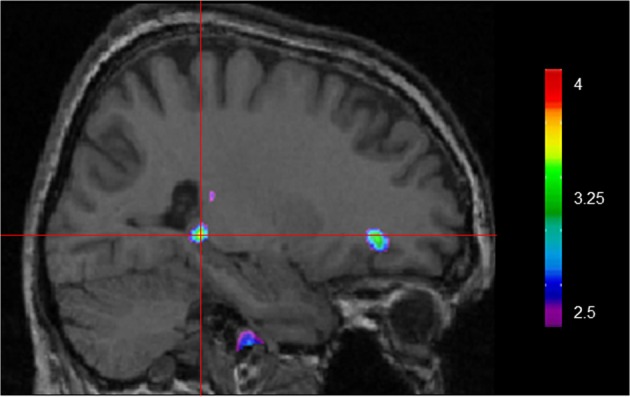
Whole brain regression analysis with scores on recombined pairs condition of CSDLT (Stage 2). Results are superimposed onto an anatomical MRI and displayed in the sagittal plane. Results show that gray matter in the hippocampus significantly co-vary with spatial navigational strategies (MNI space coordinates *x* = 26.0, *y* = −37.8, *z* = −3.2; *t* = 3.53, *p* < 0.0005).
